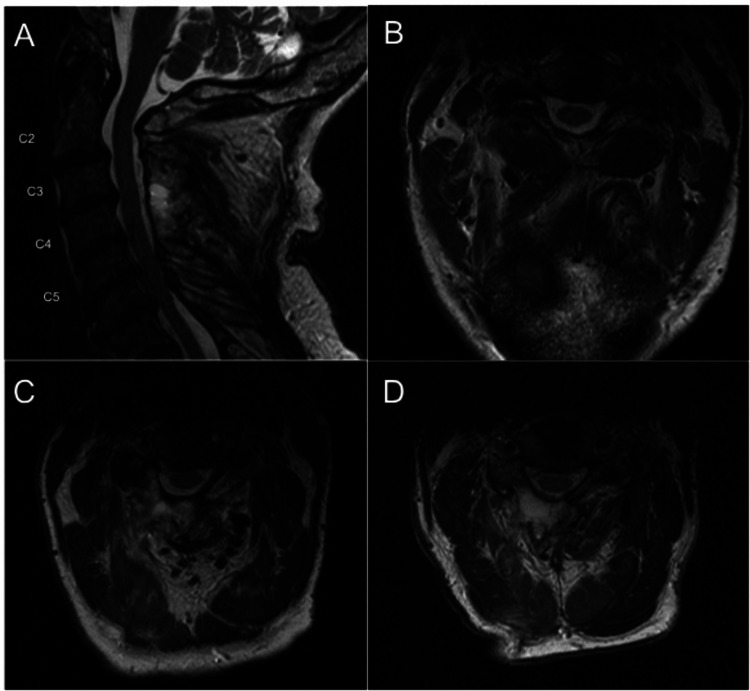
(A) Mid-sagittal T2-weighted MRI of the cervical spine status post three-level decompression with axial views at (B) C2-3, (C) C3-4, and (D) C4-5.
